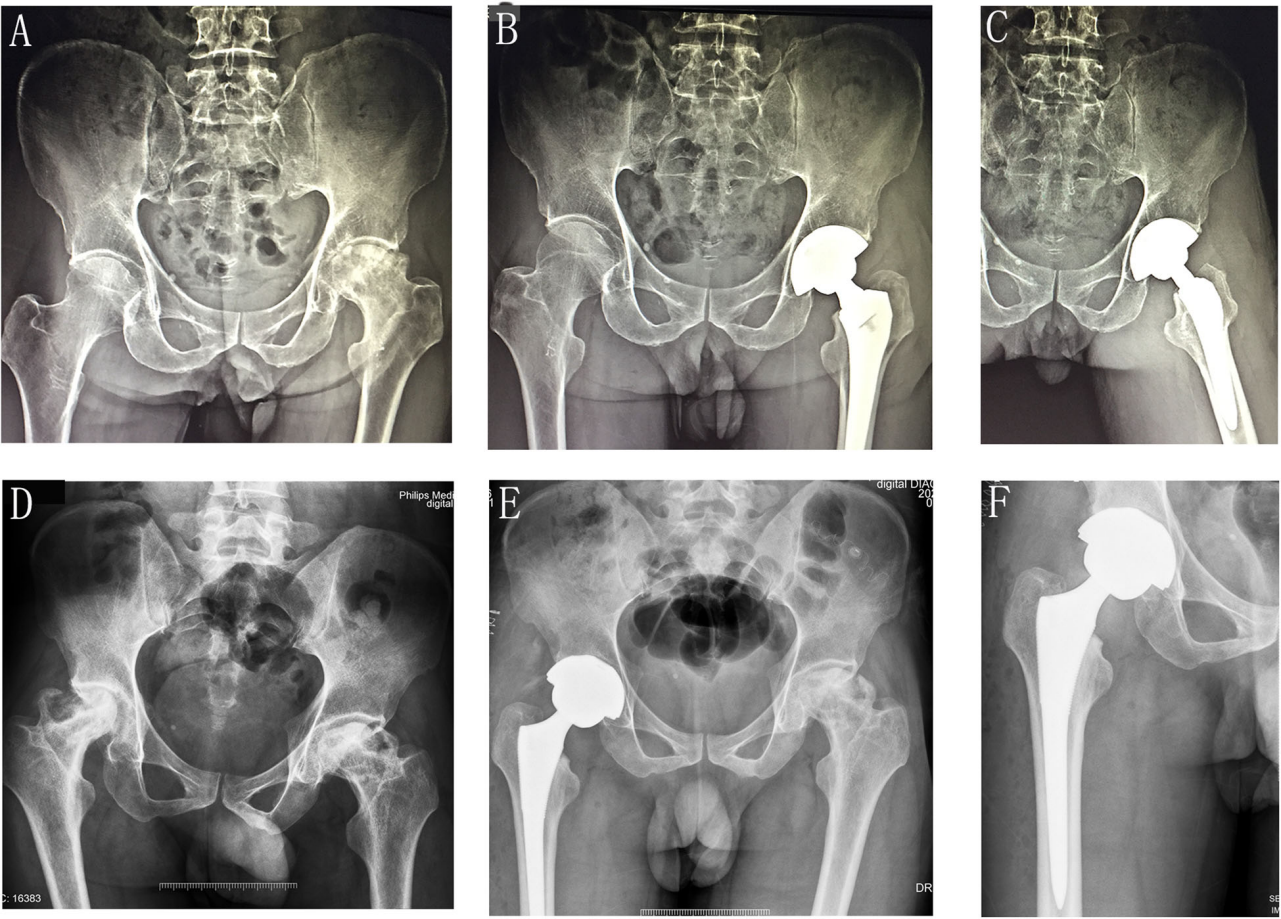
a–c Radiographic for patients undergoing SuperPath (a preoperative image for a necrosis of femoral head, left hip; b, c postoperative image). d–f Radiographic for patients undergoing PLTH (d preoperative image for a necrosis of femoral head, right hip; e, f postoperative image)
